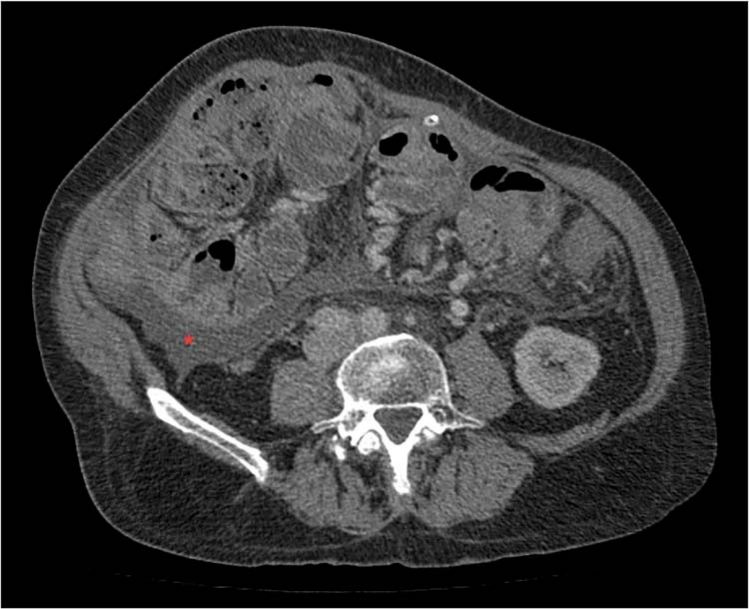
Portal phase transversal section image on CT showing membrane-like structure and loculated ascites (asterisk).
